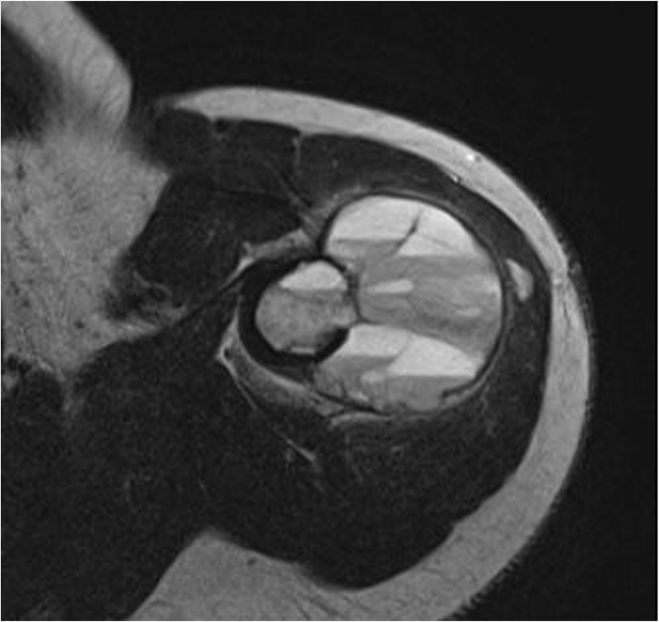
Repeat magnetic resonance imaging 6 weeks after the initial one, showing considerable growth of both the intraosseous as well as the soft tissue component of the aneurysmal bone cyst (approximately 30% and 300% respectively).
